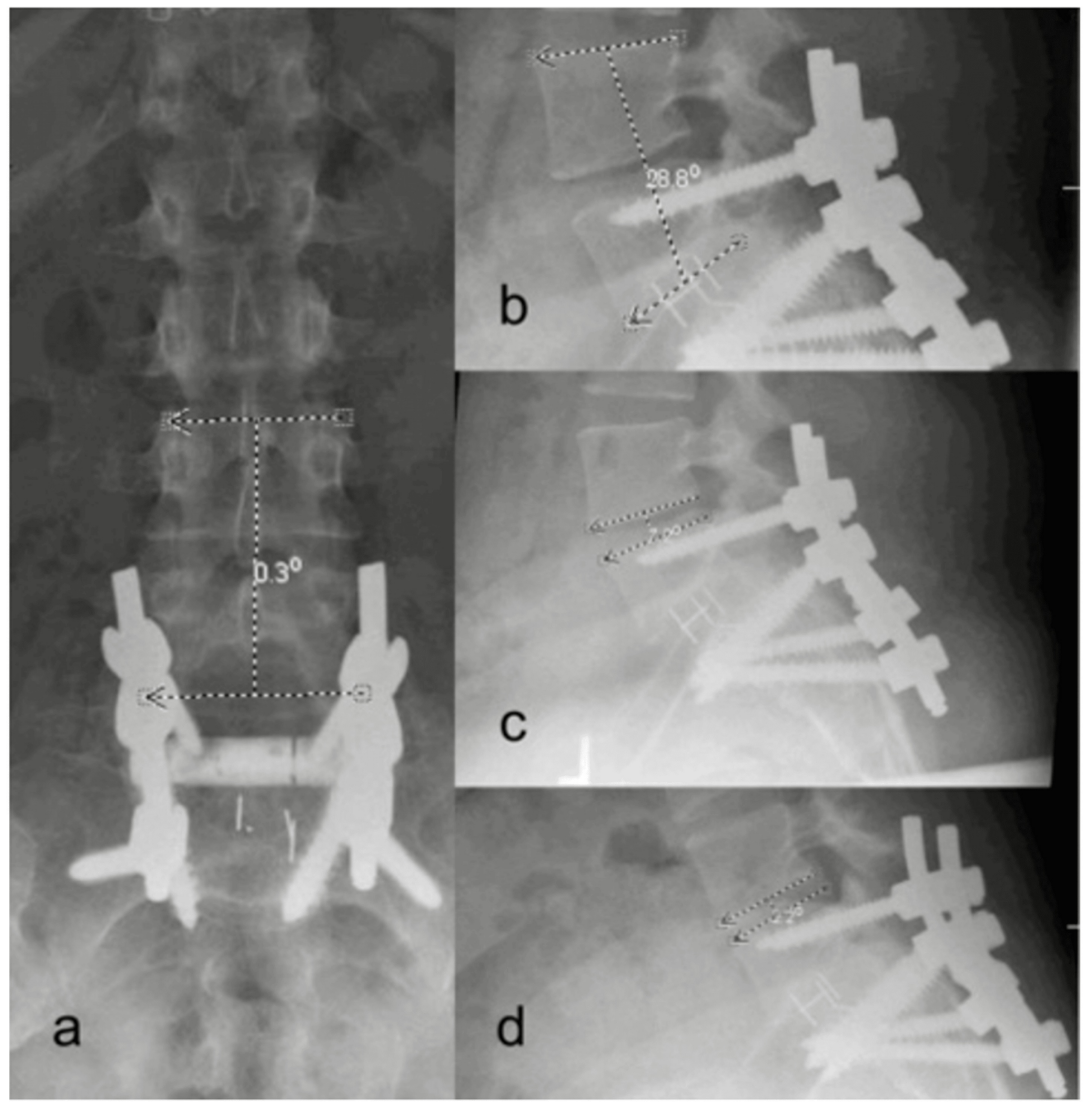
Postoperative measurements on lateral standing X-ray. (a) Anterior-posterior view. (b-d) Lateral view Source: Image: © 2024, Seattle Science Foundation. Used with permission from institution solely for this manuscript
